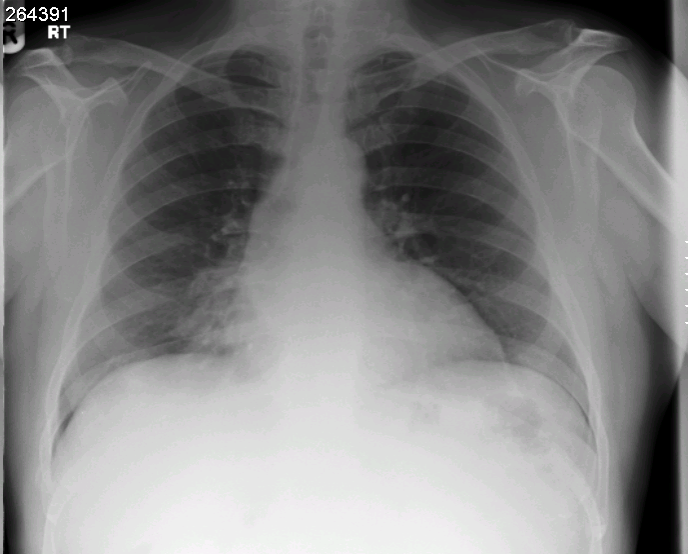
Chest radiograph showing a soft lobular paracardiac shadow in right pericardiophrenic angle.
